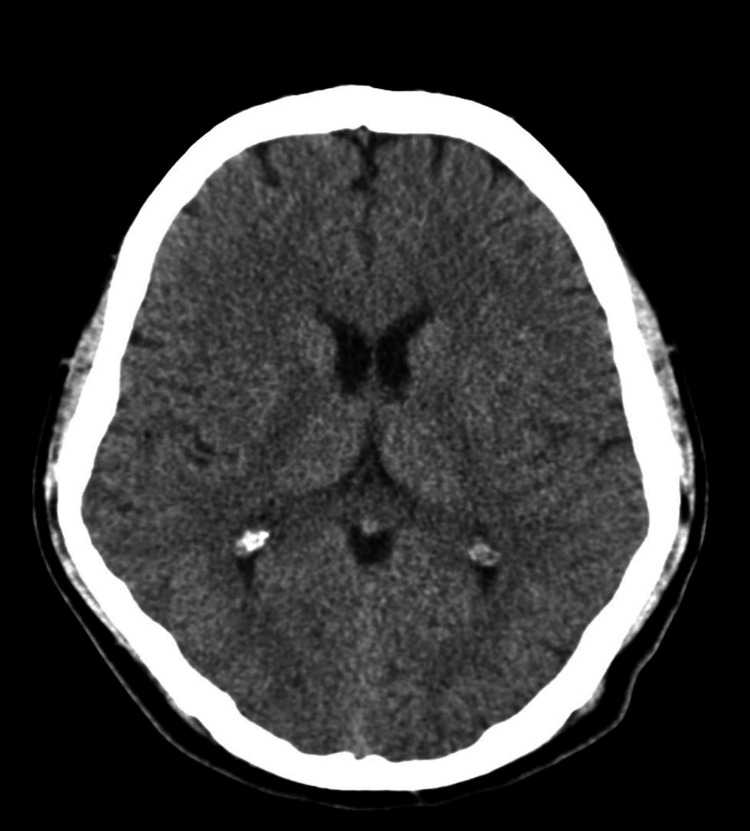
NCCT brain The NCCT brain was normal, with a preserved caudate nucleus. NCCT: non-contrast computed tomography.
